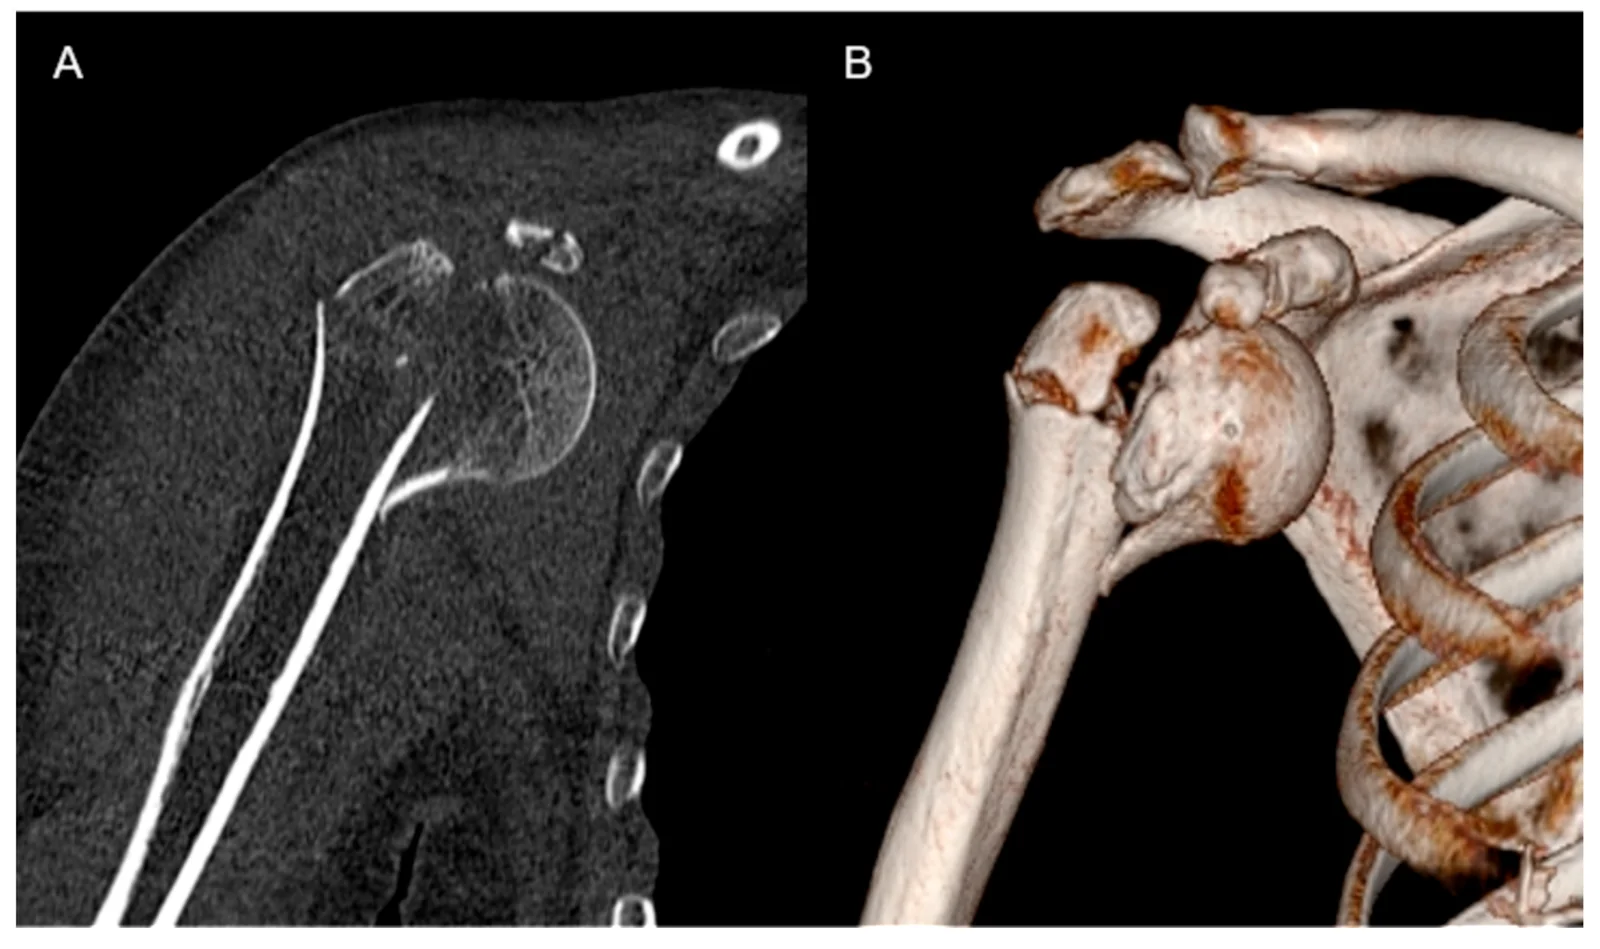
CT images of the right shoulder in the same patient as shown in Figure 6. Coronal multiplanar reconstruction (MPR) (A) and volume-rendered (VR) (B) images clearly demonstrated a comminuted fracture of the right humeral head and neck with dislocation of the right shoulder joint. The findings on the preceding shoulder radiographs were concordant with the CT findings.
